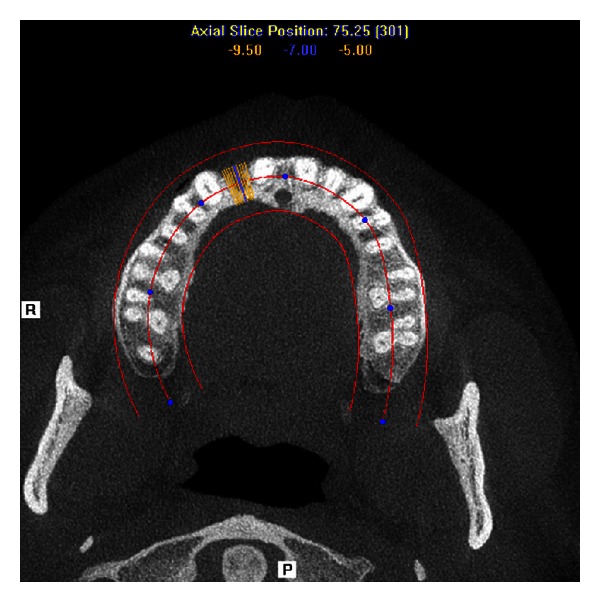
Preoperative axial section on CBCT. Decreased labio-palatal thickness of edentulous area may be noted.
